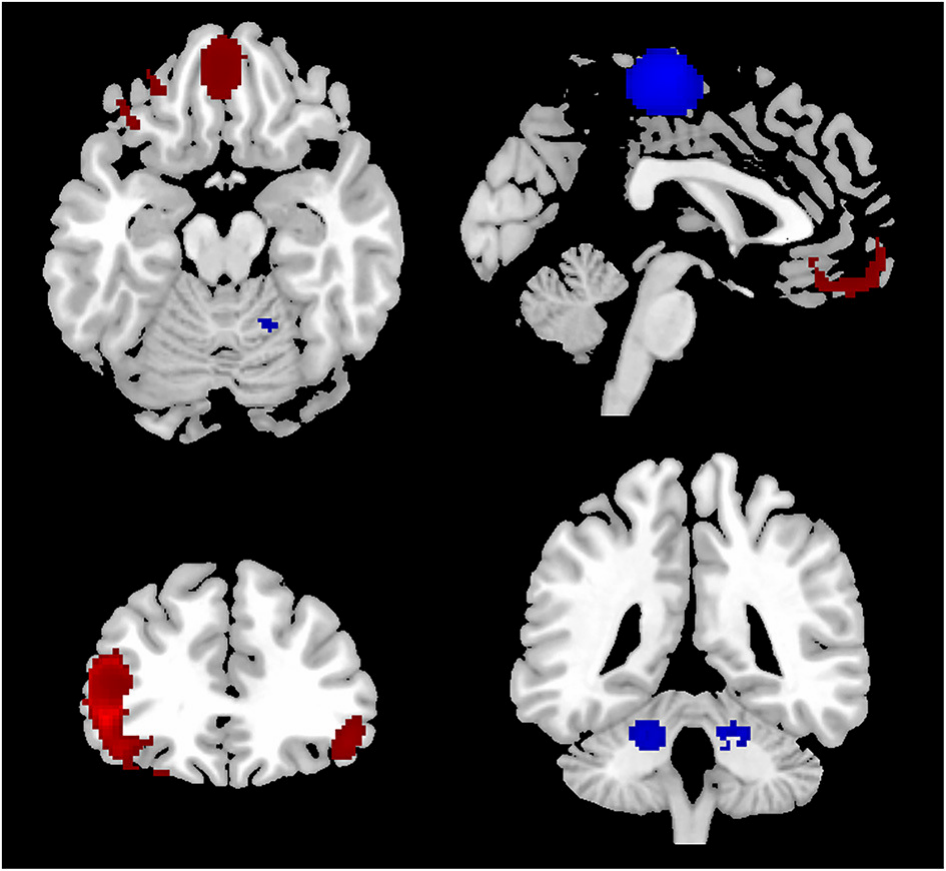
Results of the meta-analysis of whole-brain ReHo studies in patients with OCD. Regions of ReHo alterations in patients with OCD relative to HCS were shown on the three-dimensional T1-weighted template images from software (MRIcroN). Higher ReHo was shown in red color while lower ReHo was displayed in blue color. HCS, healthy control subjects; OCD, obsessive-compulsive disorder; ReHo, regional homogeneity.
